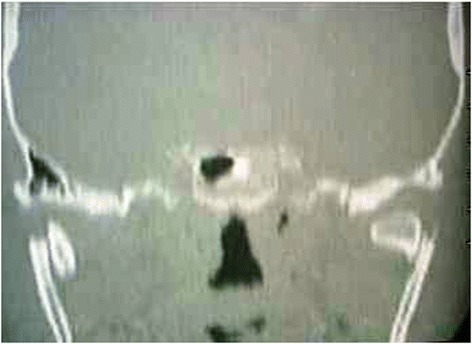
CT scan that indicat surgical treatment.
